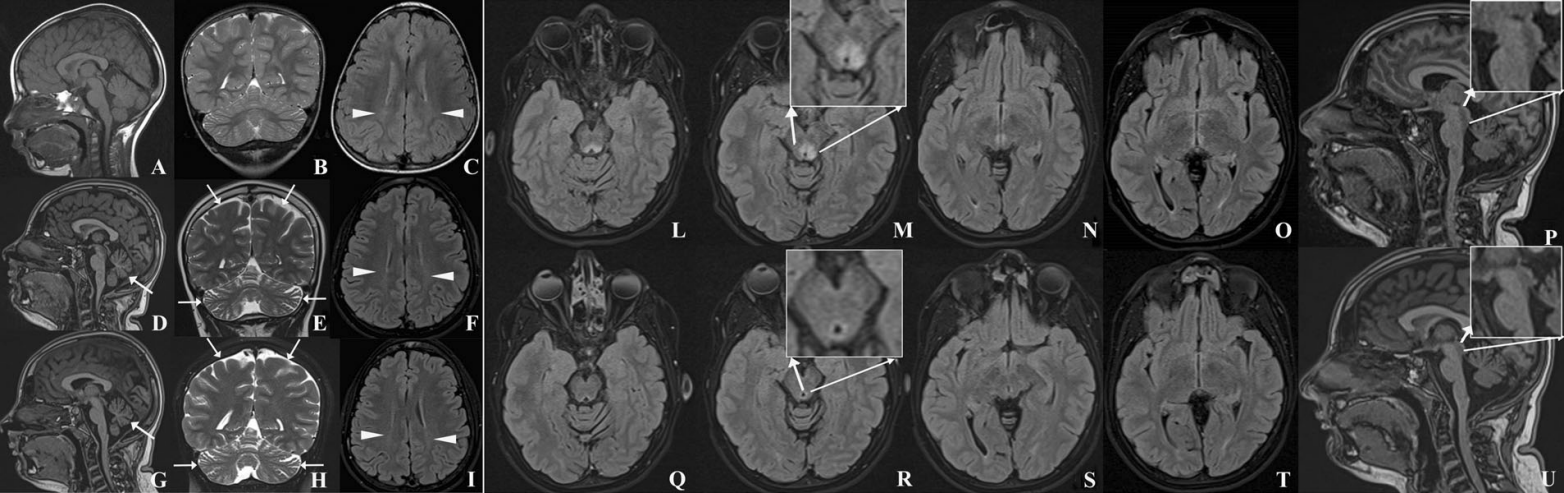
A–I. Brain MRI sagittal T1‐weighted (A, D, G), coronal T2‐weighted (B, E, H) and axial FLAIR (C, F, I) showing progressive cerebro‐cerebellar atrophy (arrows) and mild bilateral posterior white matter hyperintensity (arrowheads) at age 4.5 (A–C), 14.5 (D–F) and 16.5 years (G–I). L–U. Brain MRI axial FLAIR (L–O, Q–T) and sagittal T1‐weighted (P, U) showing Wernicke‐like pattern (magnifications of mesencephalic region) during acute ophthalmoplegia (L–P), with resolution at 2‐year follow‐up (Q–U).
